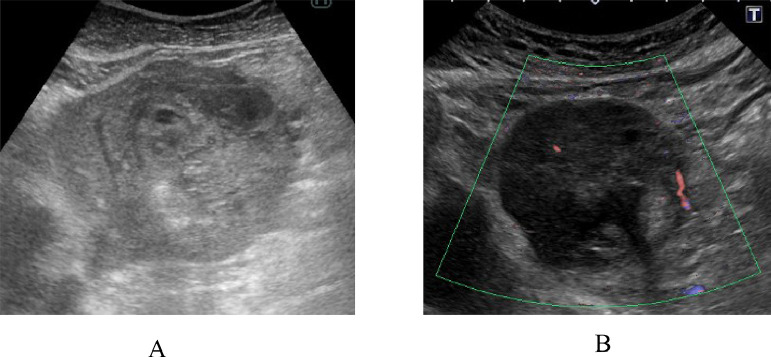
Ultrasonography of the hepatocellular carcinoma (HCC) after trans-arterial chemo-embolization (TACE). (A) Ultrasonography 6 days after TACE showed an oval mass with relatively high internal echoes. (B) Ultrasonography 48 days after TACE showed marked reduction of the tumor size, conversion of the internal echoes from high to low, and intratumoral blood flow.
